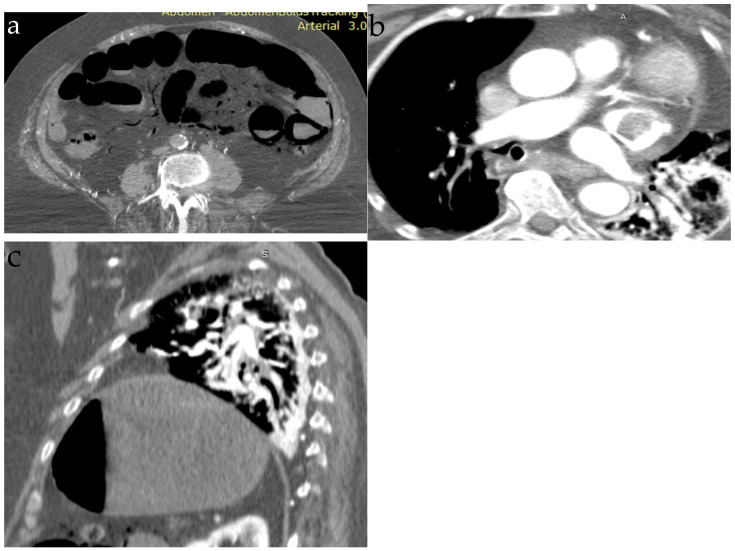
Thoraco-abdominal CT; (a) arterial acquisition with air within the branches of the superior mesenteric artery; (b,c) segmental pulmonary embolism, nonspecific (from the collection of dr. Fotea Vasile).
